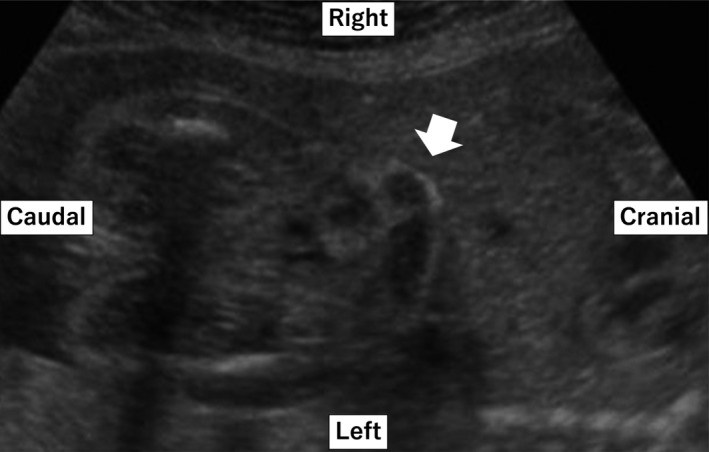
Coronal view of prenatal ultrasound at 24 wk gestation showing hyperechogenic, mildly dilated bowel (arrow)
